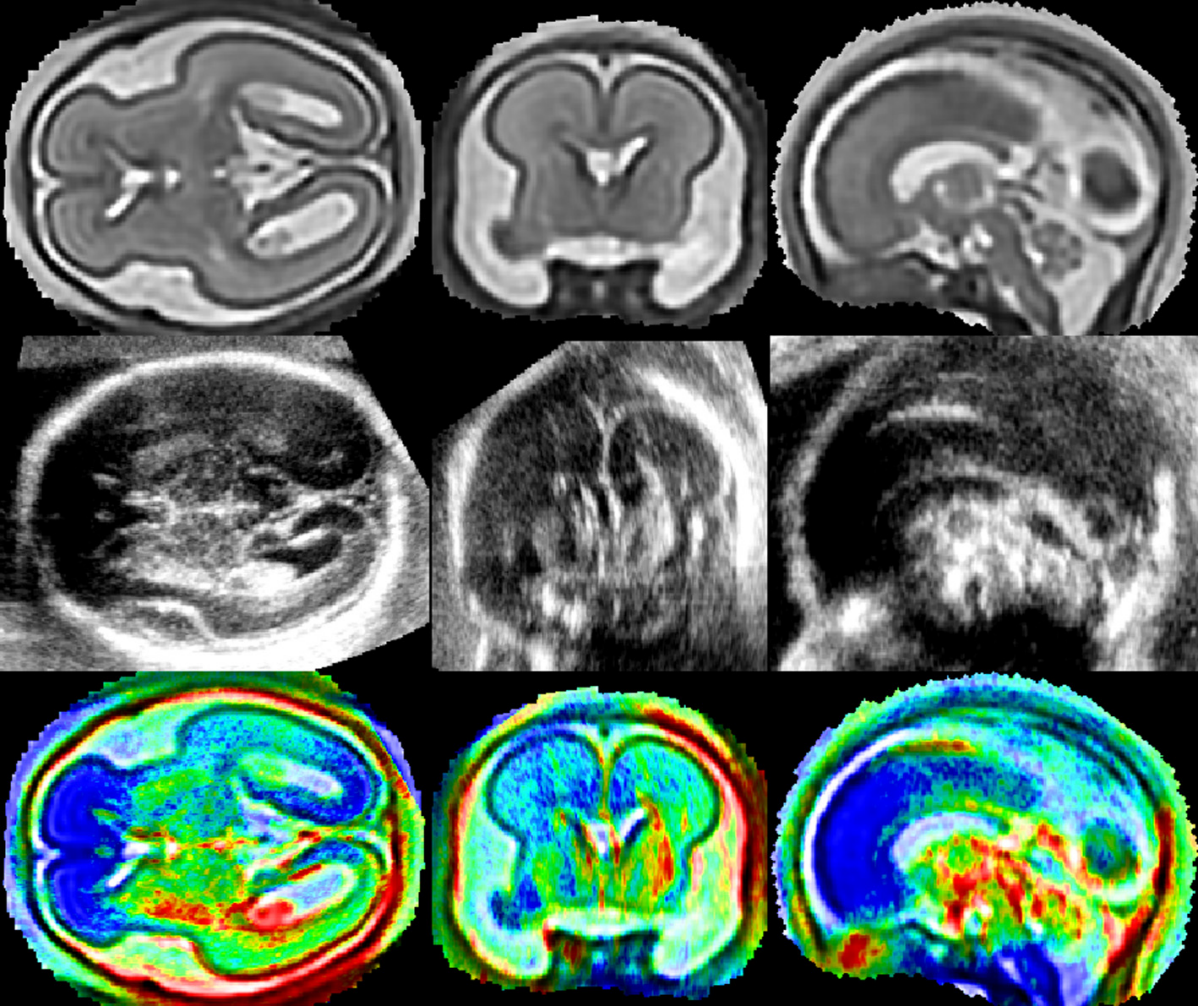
Alignment of a subject from the younger dataset with the MR template in transversal (first column), coronal (second column) and sagittal (third column) view. Top row: MR image. Middle row: US image aligned with the MR image. Bottom row: Superposition of the US image (rainbow colour-coding) over the MR image.
